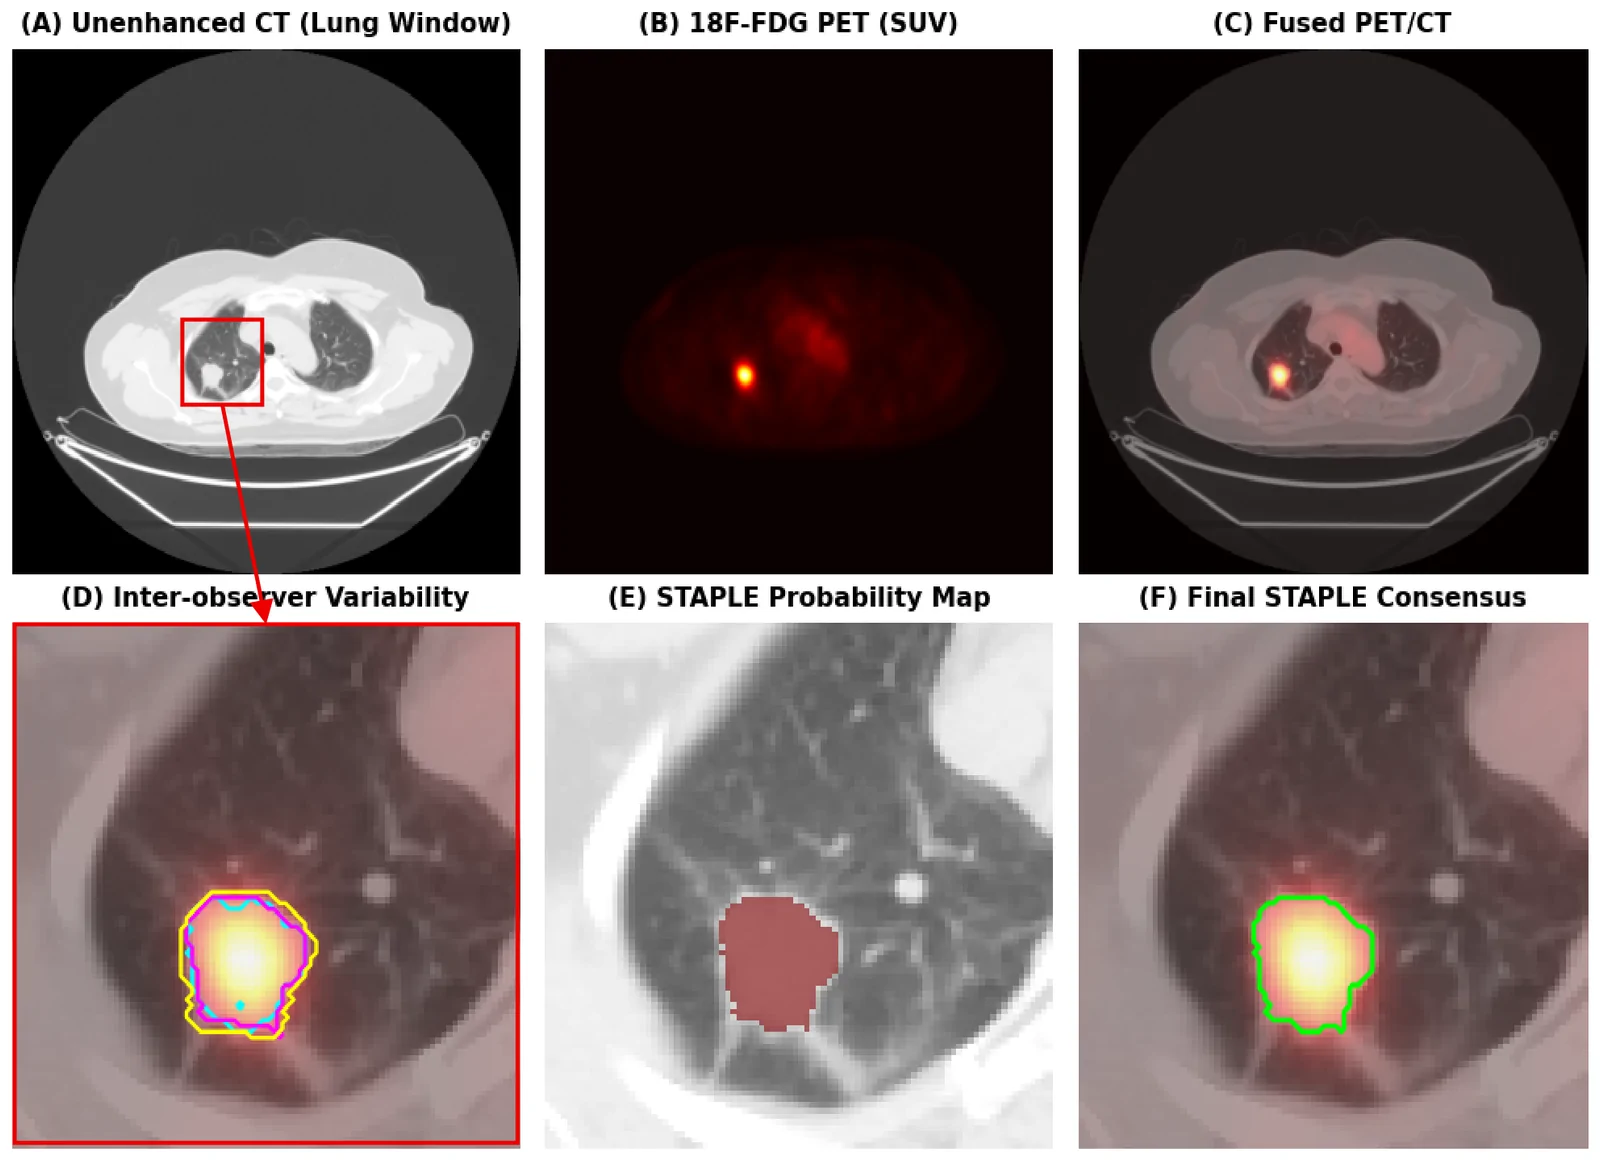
Visualization of multimodal PET/CT data and the ground-truth generation process. (A) Unenhanced CT; (B) ^18^F-FDG PET (SUV map); (C) Fused PET/CT; (D) Inter-observer variability among three experts; (E) STAPLE probability map; and (F) Final probabilistic consensus used as the reference standard.
